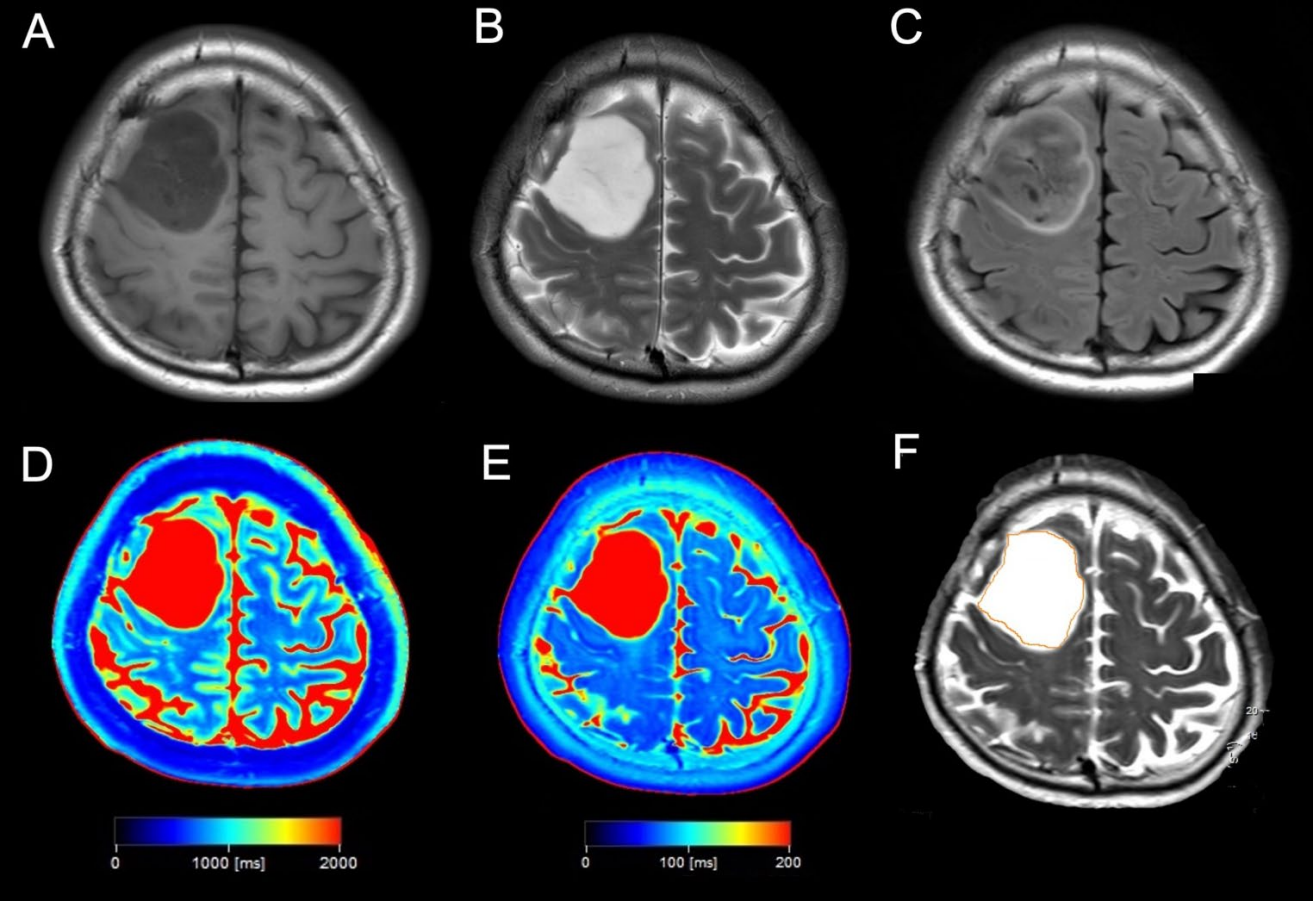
A case of a 42-year-old man with astrocytoma, IDH-mutant, grade 2. (A) T1-weighted imaging shows a homogeneous hypointense tumor in the right frontal lobe. (B) T2-weighted imaging shows a homogeneous hyperintense tumor in the right frontal lobe. (C) Fluid-attenuated inversion recovery showing signal suppression in the central tumor lesion with a hyperintensity peripheral rim, indicating a positive T2-FLAIR mismatch sign. (D, E) T1 (D) and T2 (E) maps showing prolonged T1 and T2 values in the tumor lesion. (F) The ROI was drawn in tumor lesion.
